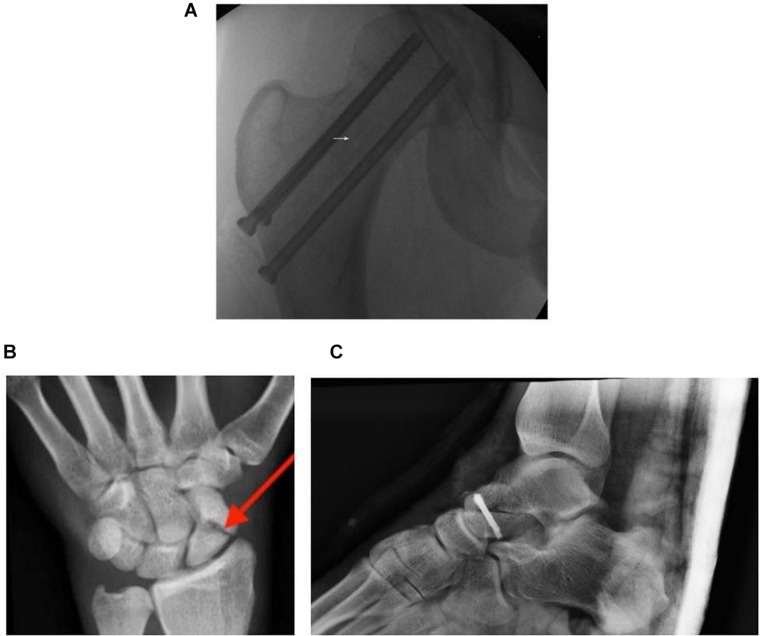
(A) An anteroposterior fluorograph of a displaced femoral neck fracture fixed with cannulated screws. The femoral neck (region around white arrow) connects the femoral head to the rest of the femur. (B) Plain radiograph of the left anteroposterior carpals of a young adult presented with a full thickness scaphoid fracture (red arrow). (C) Post-operative plain radiograph of a talus presented with a fracture. Adapted from Patel (2012), Li et al. (2013), and Kim and Yanuck (2018).
